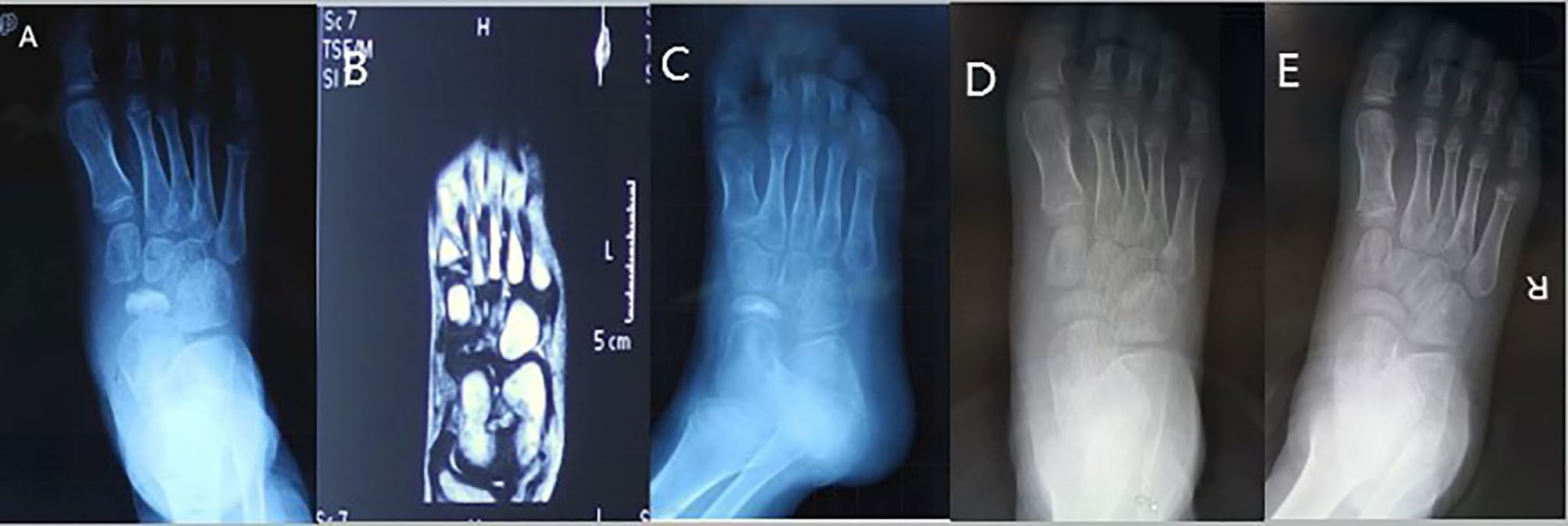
Case 1 radiography changes of the navicular after vascular bundle implantation. (A) Pre-operation X ray showed navicular bone sclerosis; (B) Pre-operation MRI showed ischemic status of the navicular bone; (C) 1 month post-operation X ray showed radiography improvement of the sclerotic navicular bone; (D) 3 months post-operation X ray; E, 6 months post -operation X ray.
